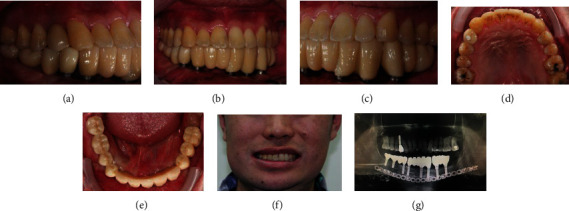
Completed dental reconstruction. (a–c) Final insertion of zirconia implant-supported fixed partial dentures and ideal occlusal plane and occlusion. (d, e) Smooth and symmetrical dental arches. (f) Photograph showing improved asymmetry of face. (g) Radiograph of the final mandible and dental reconstruction.
